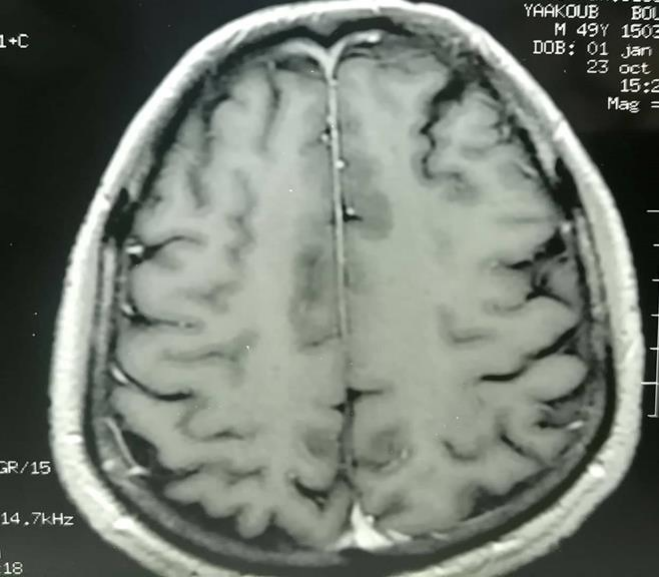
Cerebral MRI revealing frontal pachymeningitis
